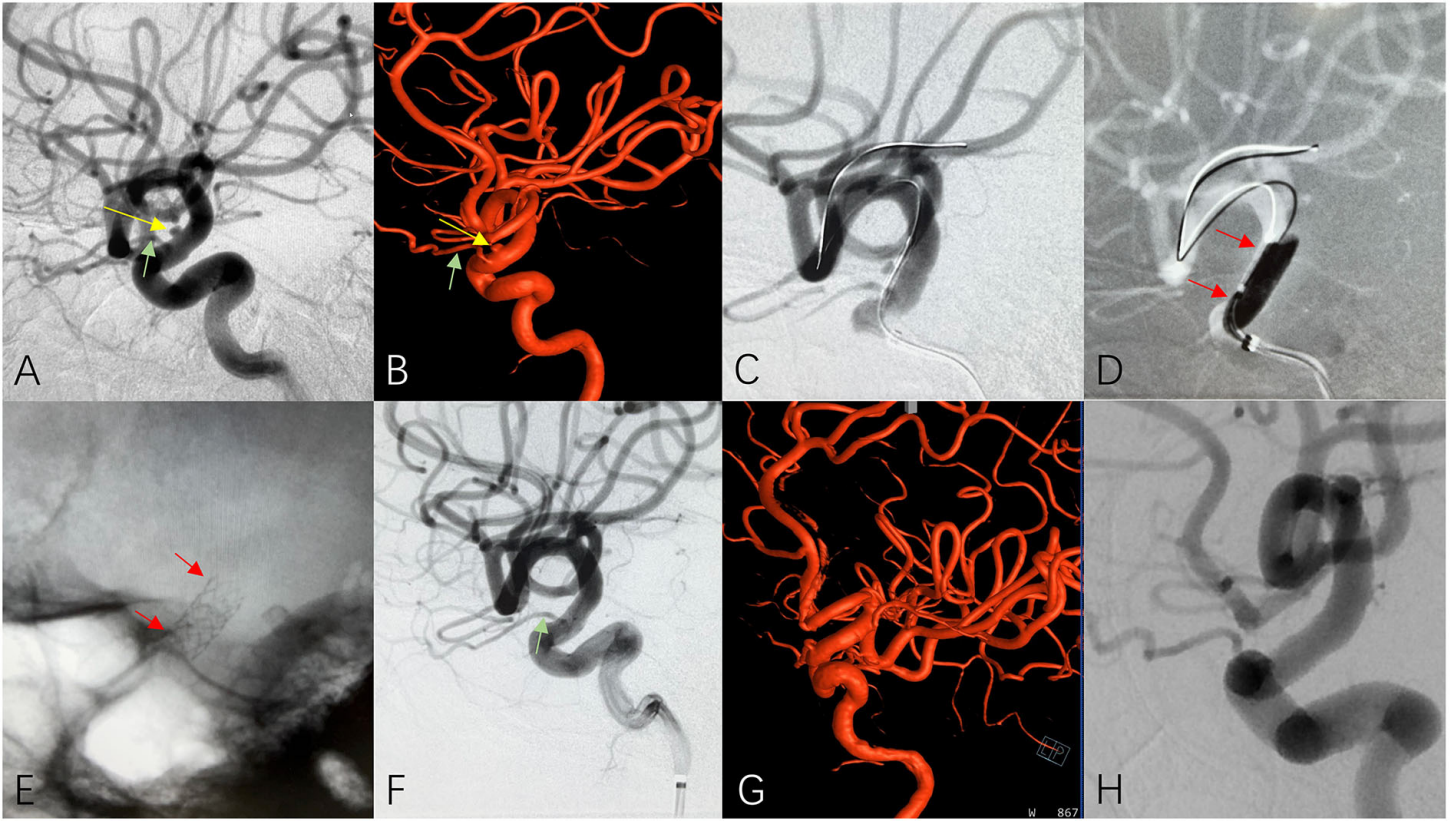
A patient with cerebral aneurysm received covered stent insertion. 2D (A) and 3D (B) cerebral angiograms reveled a unruptured tiny cerebral aneurysm (yellow arrow) and adjacent ophthalmic artery (green arrow) at the C6 segment of ICA. A covered stent (3.5 × 10 mm) was delivered (C, red arrows) released at the diseased parent artery (D, red arrows) and the plain film clearly showed well opened covered stent after deployment (E, red arrows). Immediate 2D (F), 3D (G) and 4-months follow up (H) cerebral angiogram confirmed complete disappearance of aneurysm and excellent patency of the ophthalmic artery (green arrow) and parent artery.
